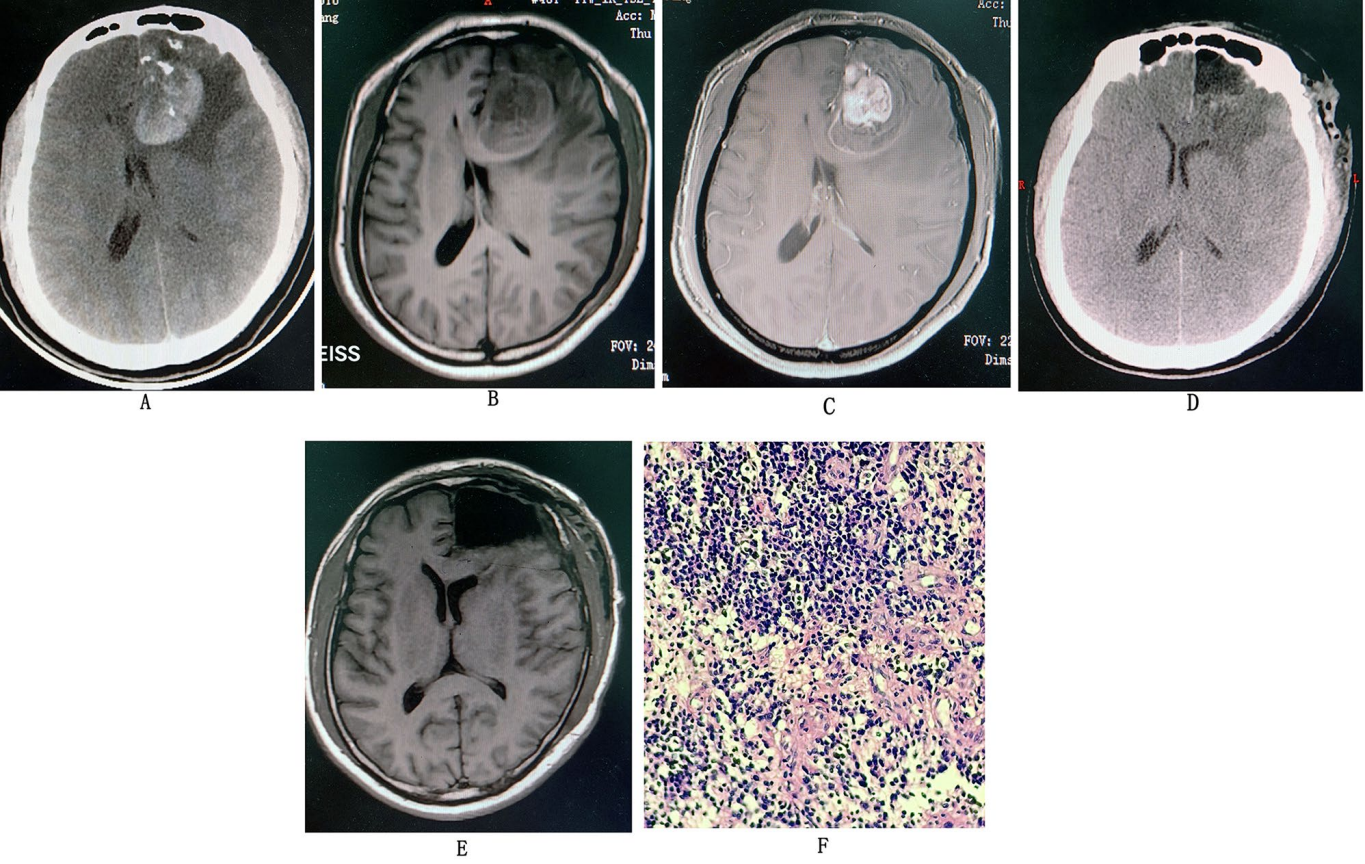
(A) Head CT after onset of symptoms in the patient shows a visible left frontal lobe space-occupying lesion with apoplectic intratumoral hemorrhage; to consider glioma complicated hemorrhage. (B) Preoperative cranial MRI T1 phase. (C) Postoperative MRI enhancement. (D) Postoperative CT of the head showing total resection of the tumor with midline gyration. (E) Postoperative MRI of the head showing the tumor is totally resected without any residue. (F) Postoperative pathology results showing anaplastic oligodendroglioma of WHO-grade 3
